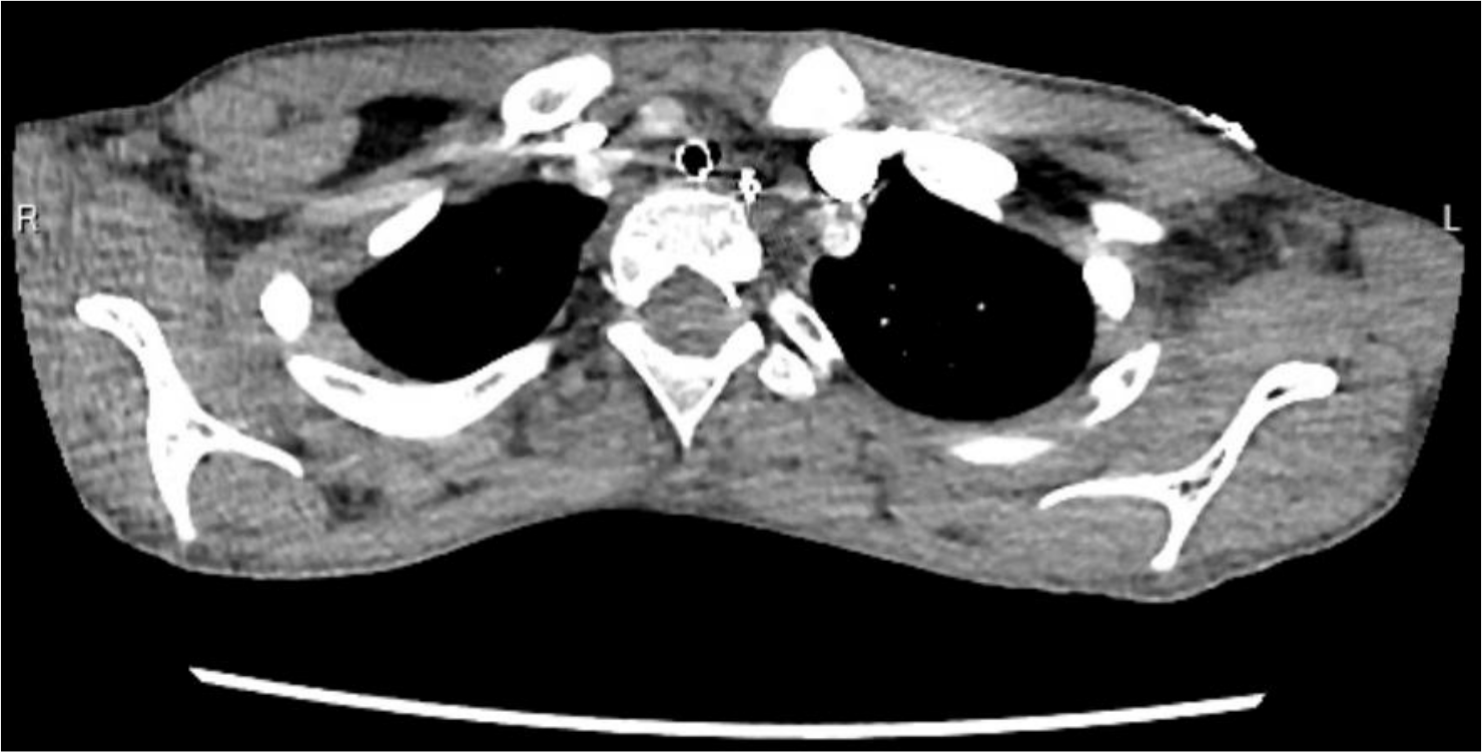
CT chest with contrast showing the innominate artery abuts the trachea causing focal compression with no air seen around the endotracheal tube.
